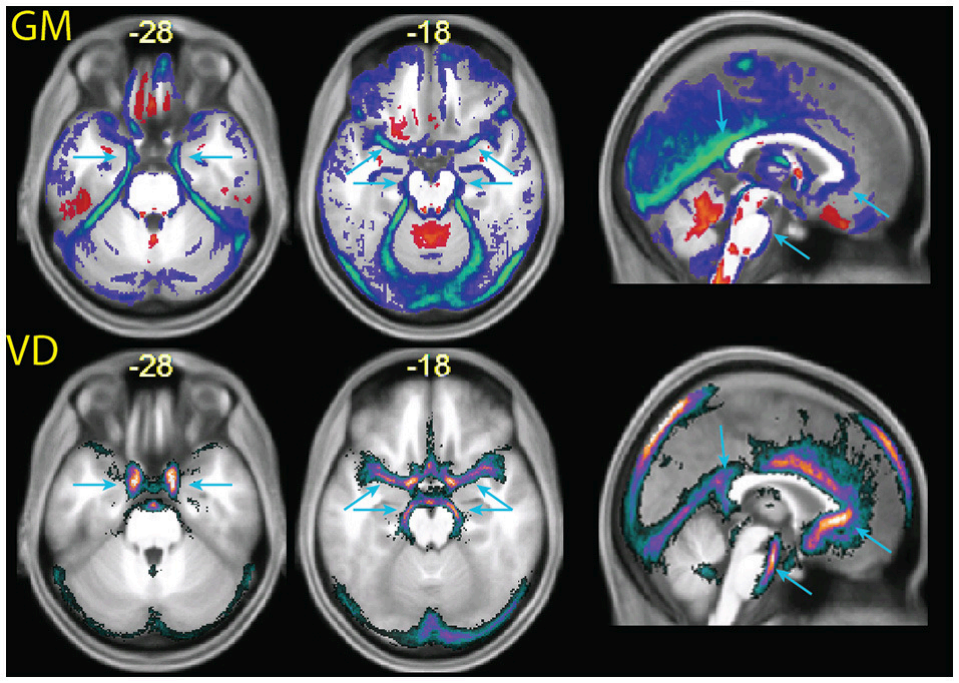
(Top row) enlarged slices from Figure 5, showing differences in the tissue probability maps for gray matter (GM) obtained with the MPRAGE+FLAIR combination relative to the MPRAGE segmentation. (Bottom row) images of vascular density (VD), obtained from a publicly available vascular density atlas from time-of-flight images segmented semimanually (Viviani, 2016). The top of the brain is not represented in these data due to missing coverage. Note that the multimodal segmentation identifies more tissue as non-gray matter than the time-of-flight based data (for example around the cerebellum in the center slice), due to the different intensity of connective tissue in the T2-weighted signal (Helms et al., 2006).
